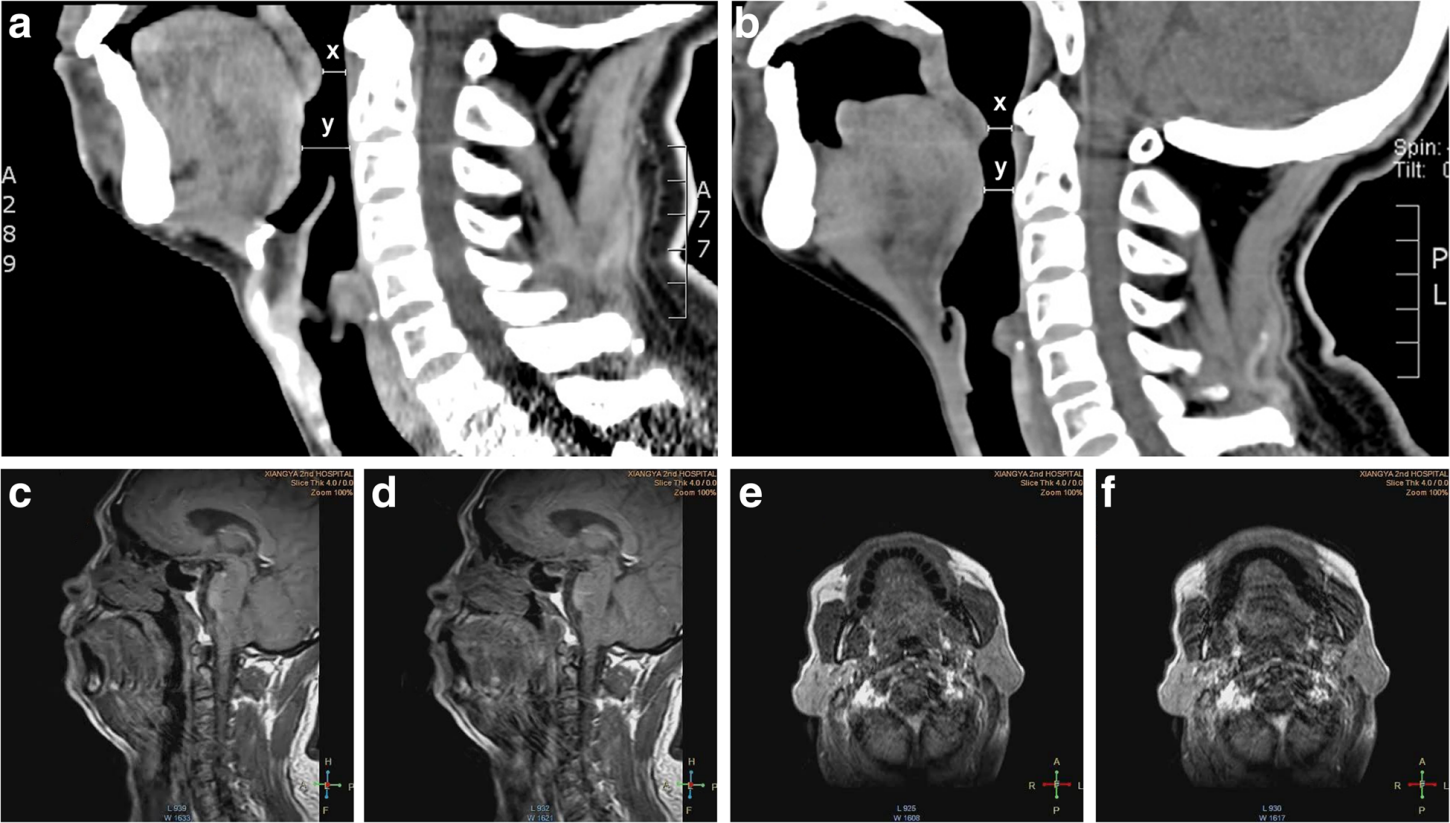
Anteroposterior diameter of retrolingual space (x) and retropalatal space (y) in CT sagittal plane of one patient undergoing SCPL before the surgery (a) and postoperation (b). Retropalatal and retrolingual space in the expiratory phase (c) and aspirated phase (d) in sagittal plane of dynamic sleep MRI; Retropalatal and retrolingual space in the expiratory phase (e) and aspirated phase (f) with one patient undergoing SCPL in transverse plane of MRI
